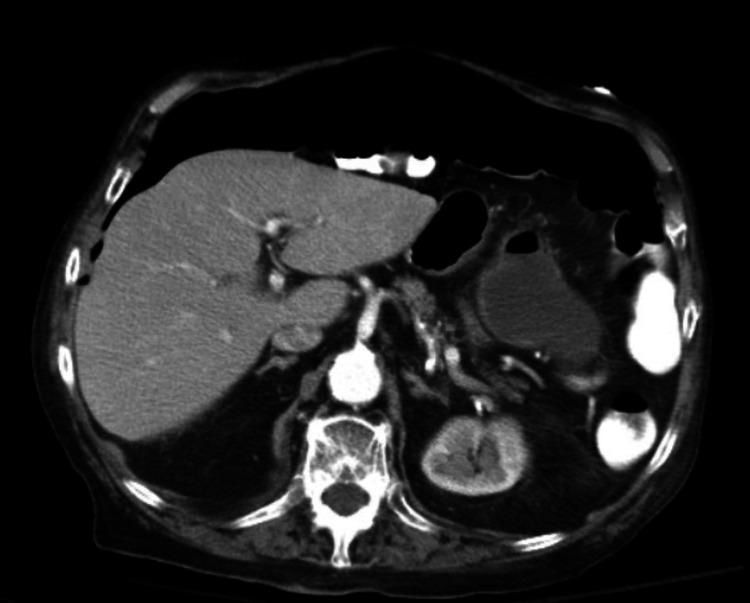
CT abdomen showing stomach having a oval 7.6 cm × 4 cm × 5 cm fluid collection with internal content debris and air bubbles along the greater curvature
